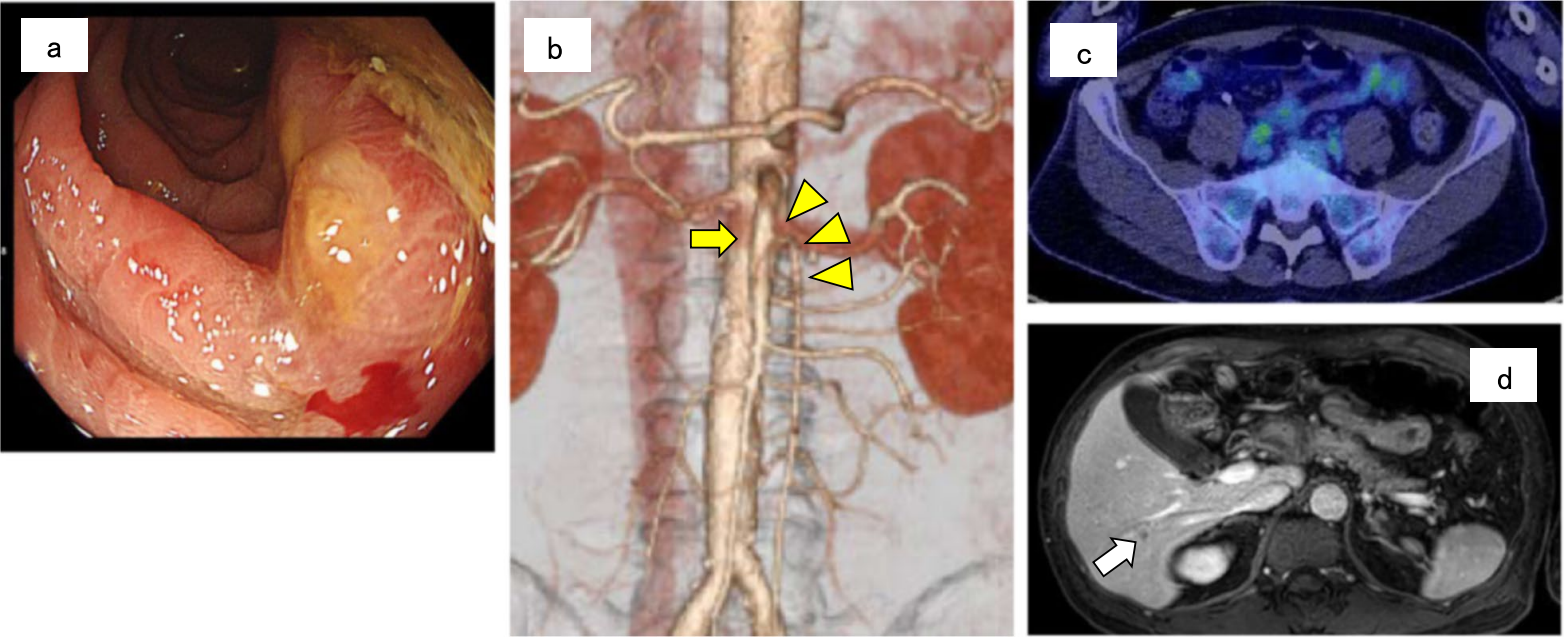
Preoperative imaging findings. a Colonoscopy showed a semi-circumscribed irregular elevated lesion in the sigmoid colon. b 3D-CT angiography showed that the IMA (yellow arrowheads) originated from the SMA (yellow arrow). c On PET-CT, there was no abnormal accumulation in the central lymph nodes around the IMA. d MRI showed findings suggestive of liver metastasis (white arrow)
